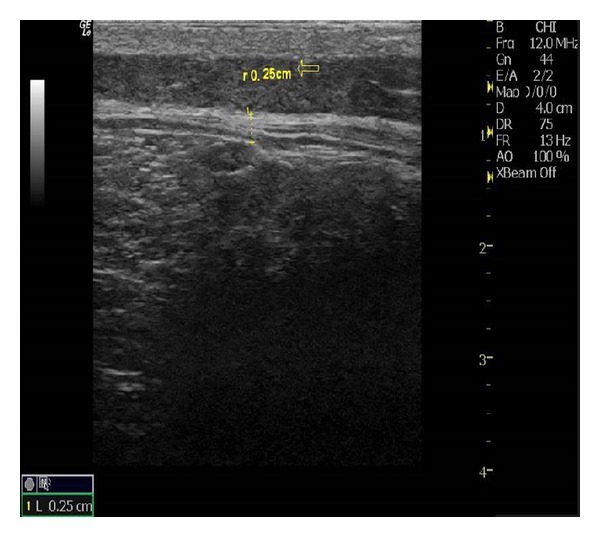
Fascia thickening under color doppler ultrasound.
